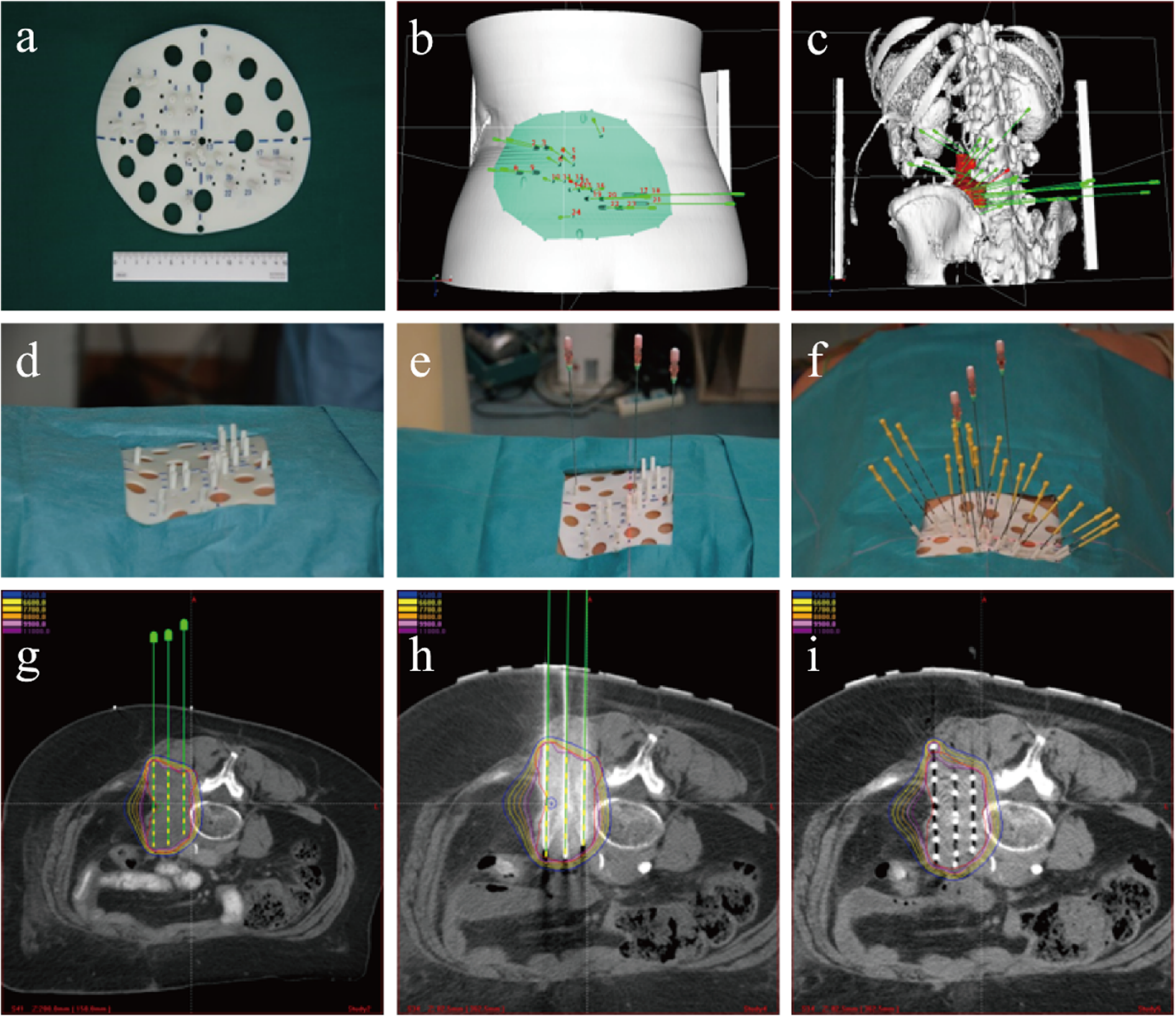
The work-flow of 3D-PNCT-assisted CT guidance RSI-BT. a 3D-PNCT. b 3D-PNCT reconstruction on CT images. c 3D model view of bony anatomy and the puncture needle distribution reconstruction. d 3D-PNCT set-up: e stable needle insertion based on preplan, f seed needle insertion, g preplan, h intraoperative real-time plan, and i postoperative plan
